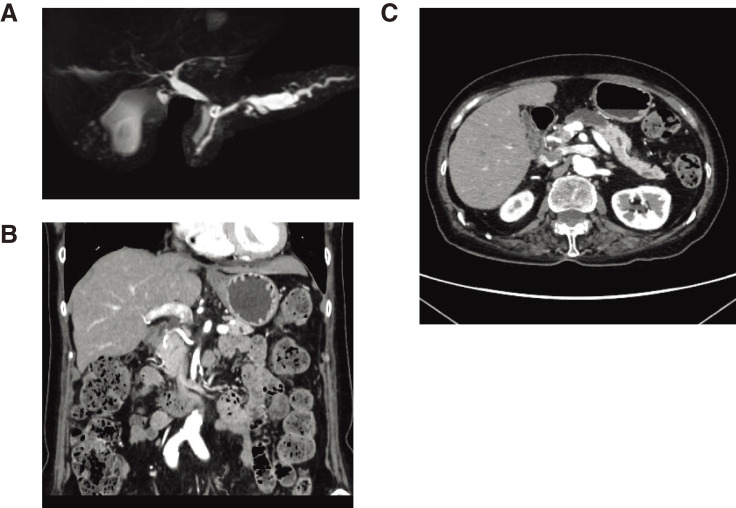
Preoperative imaging findings. (A) Magnetic resonance cholangiopancreatography shows the dilation of the main pancreatic duct (MPD) and the meandering MPD. (B) Computed tomography (CT) shows the replaced common hepatic artery (rCHA) surrounded by the pancreas. (C) CT shows that the MPD runs behind the rCHA.
